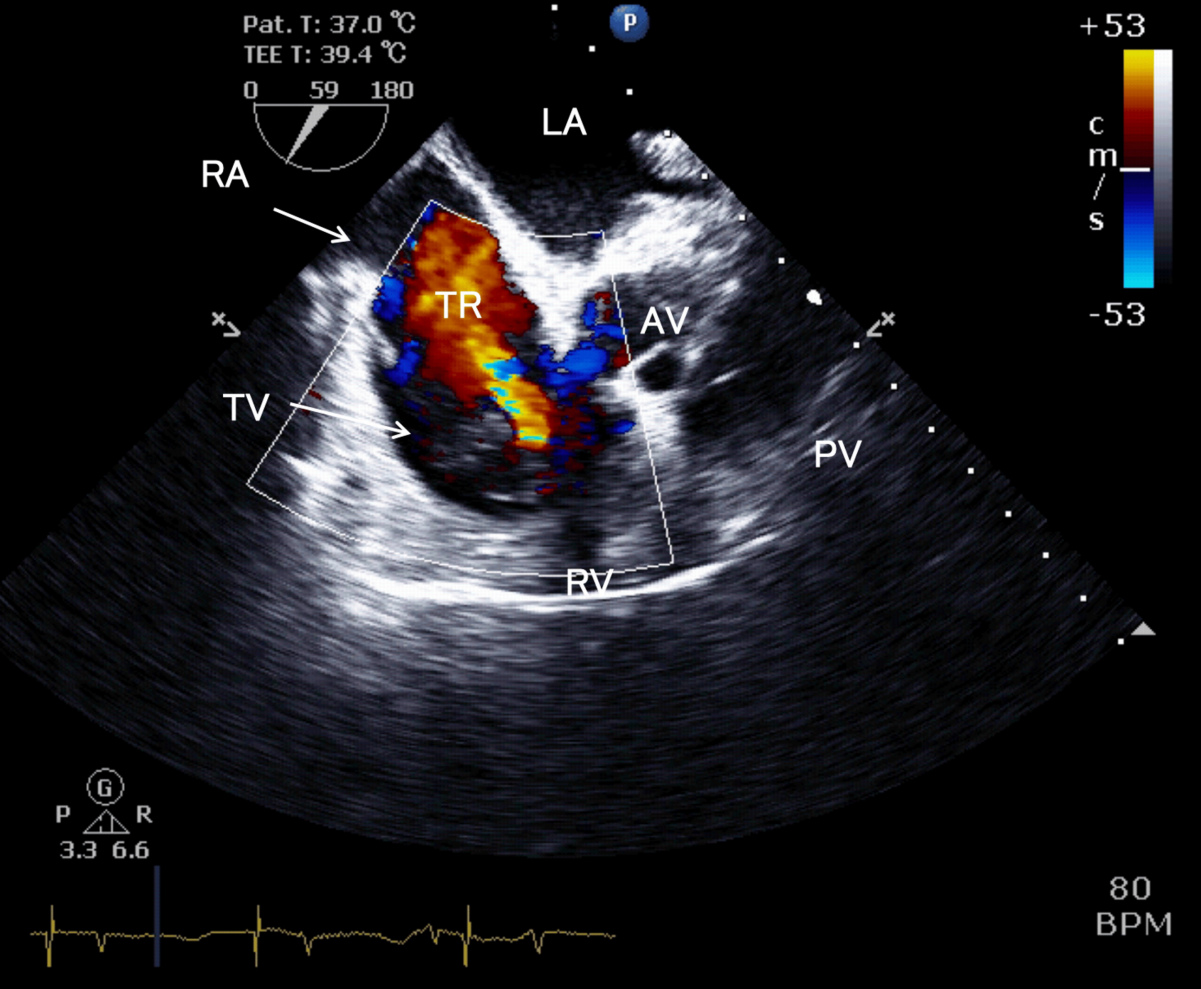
Post-procedural transesophageal echocardiography, right ventricular inflow-outflow view This is a right ventricle inflow-outflow view image taken after tumor resection and weaning from cardiopulmonary bypass. After removing the tumor that had impacted the tricuspid valve, moderate or severe tricuspid regurgitation was observed. Despite the tricuspid regurgitation, the right ventricular function remained good, unchanged from the preoperative state. TV, tricuspid valve; RA, right atrium; RV, right ventricle; TR, tricuspid regurgitation; LA, left atrium; AV, aortic valve; PV, pulmonary valve
